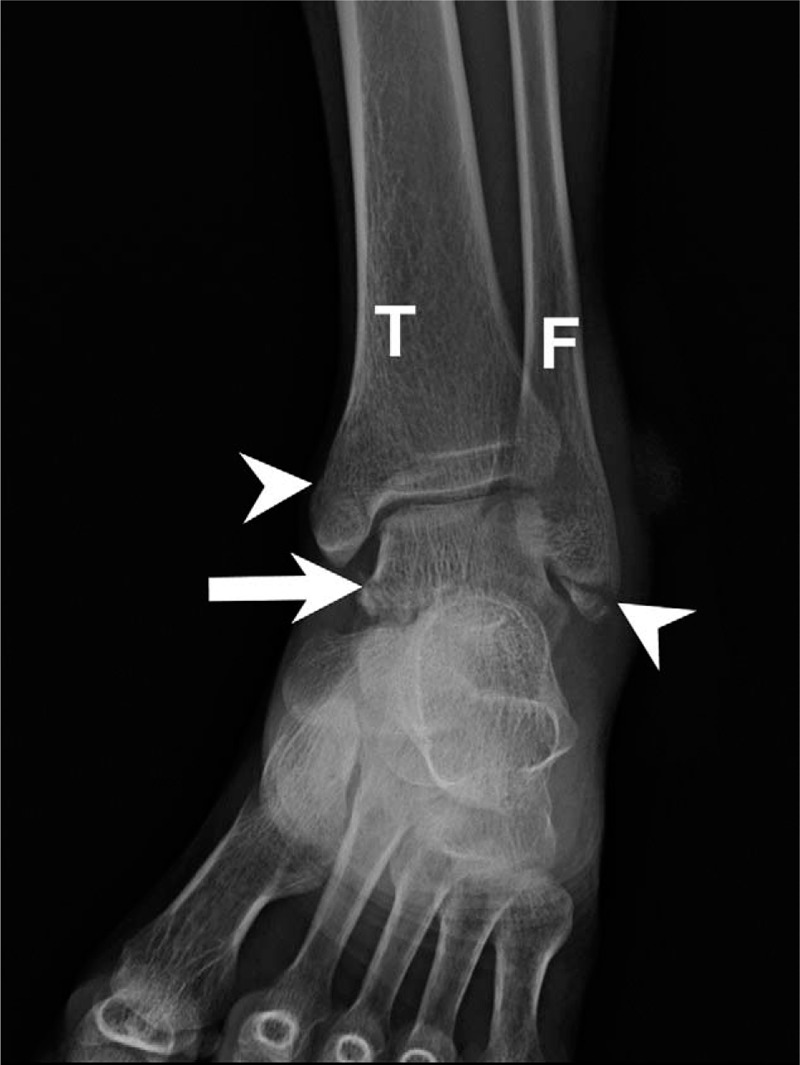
Fractures resulting from an accident in a 57-yr-old patient. Talar fracture with bimalleolar fracture diagnosed on an ankle anteroposterior plain film. Arrow = talus, Arrowhead = malleolar fracture site; F = fibula; T = tibia.
